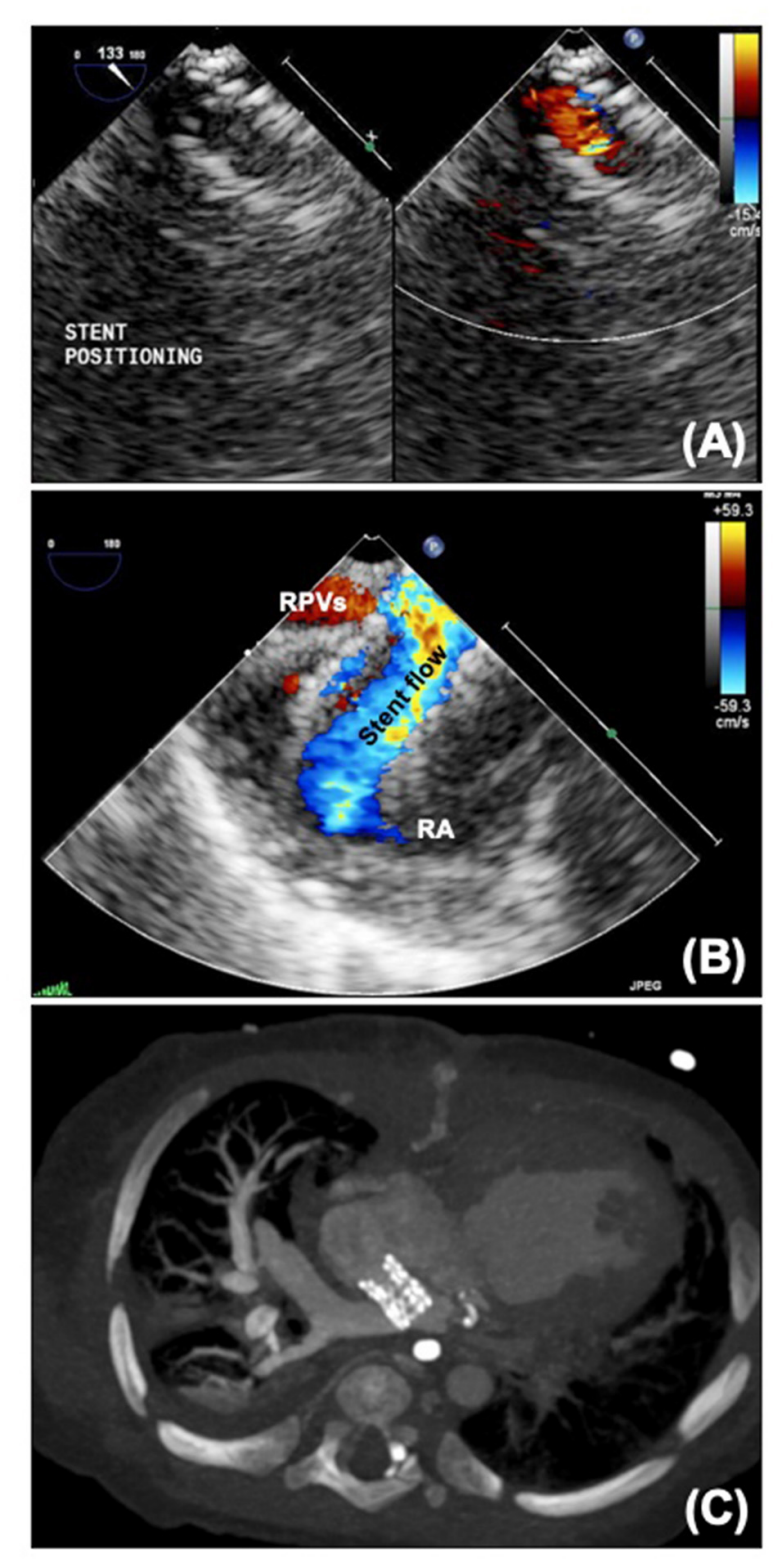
(A) Micro transoesophageal echocardiographic guidance of atrial septal stent. (B) Jailed pulmonary veins seen on echo. (C) CT scan showing atrial septal stent with dilated right pulmonary veins. RA, Right atrium; RPVs, right pulmonary veins.
